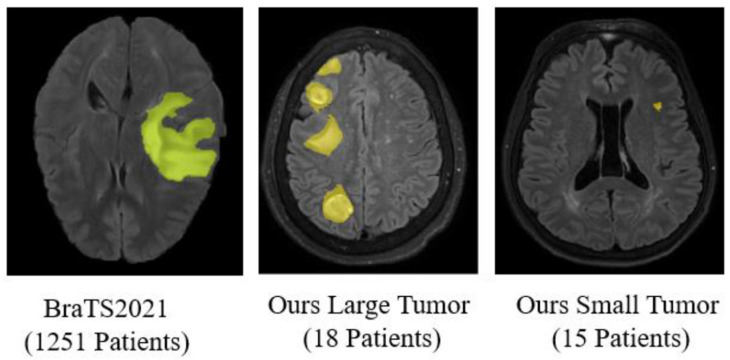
MRI Images of large and small tumors.
